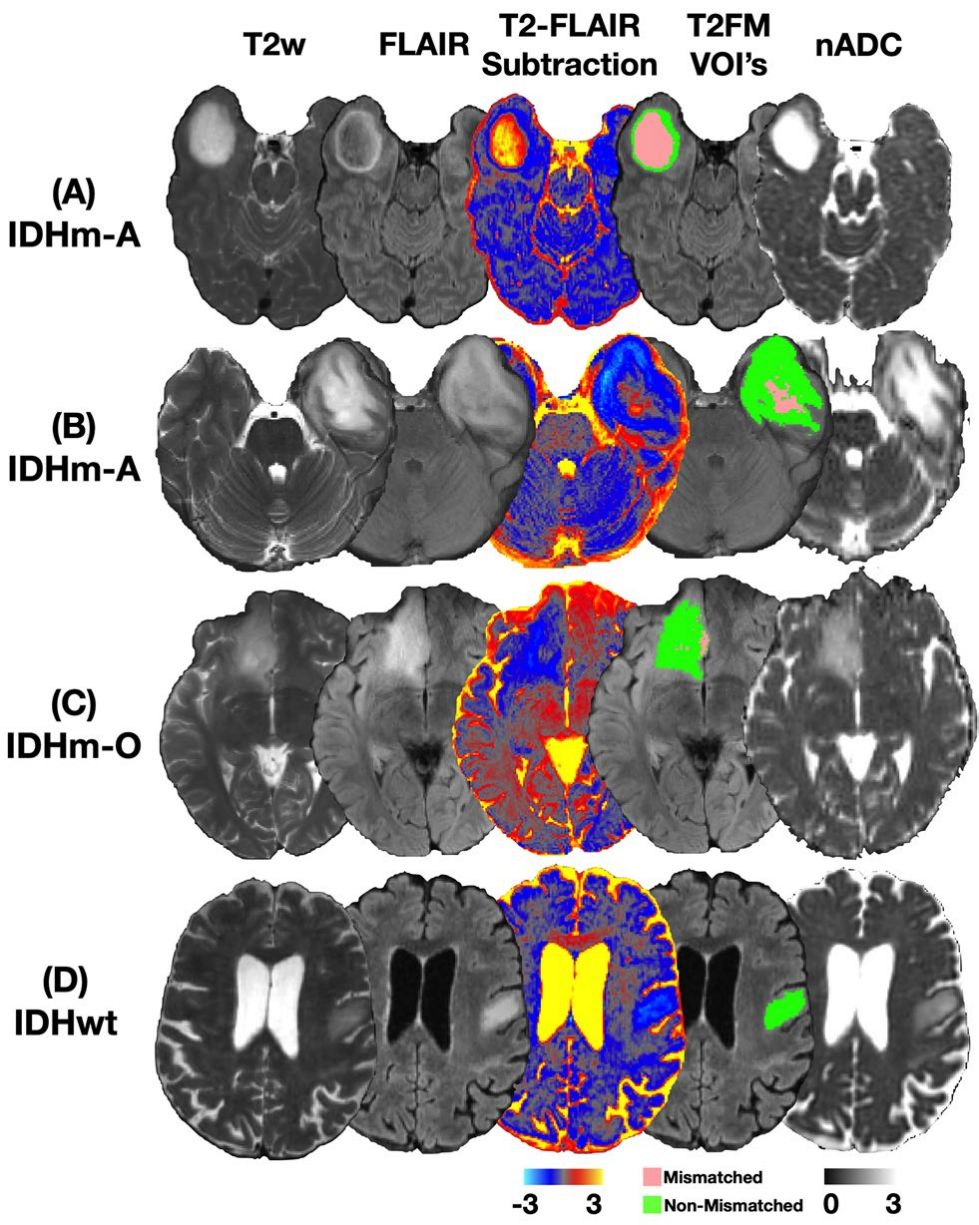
Four representative cases with quantitative T2-FLAIR subtraction and nADC maps. (A) Patient A is a 36-year-old male diagnosed with IDH-mutant astrocytoma (IDHm-A) with 3.02 nADC and 53.5% T2-FLAIR mismatch volume (T2FM-volume) (“mismatched”). (B) Patient B is a 36-year-old female diagnosed with IDHm-A with 2.15 nADC and 10.9% T2FM-volume (“non-mismatched”). (C) Patient C is a 27-year-old female diagnosed with IDH-mutant oligodendroglioma (IDHm-O) with 1.78 nADC and 6.8% T2FM-volume. (D) Patient D is a 75-year-old female diagnosed with IDH-wild type (IDHwt) glioma with 1.62 nADC and < 1% T2FM-volume. Tumor segmentation volumes of interests (VOIs) denoting T2FM subregions (pink) and T2-FLAIR non-mismatch (T2FNM) subregions (green) are shown. IDHm-A = isocitrate dehydrogenase mutant astrocytoma; IDHm-O = isocitrate dehydrogenase mutant oligodendroglioma; IDHwt = isocitrate dehydrogenase wild type glioma; T2FM = T2-FLAIR mismatch; nADC = normalized apparent diffusion coefficient
